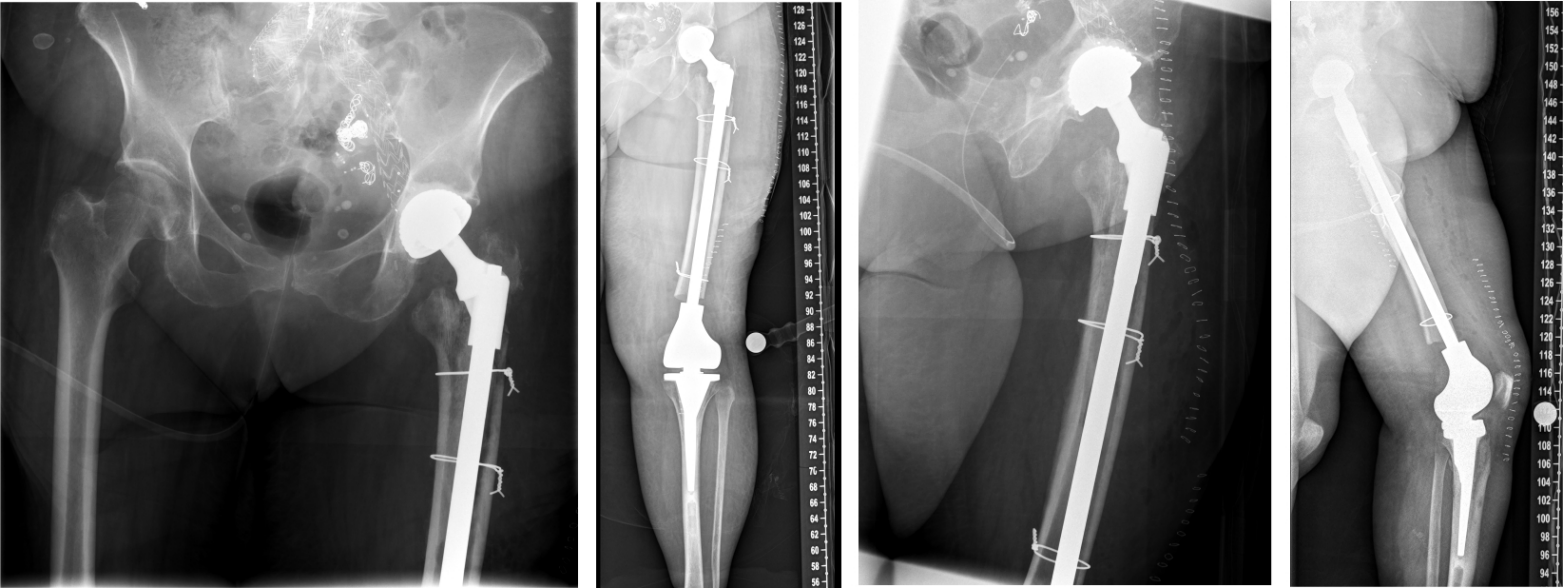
The postoperative radiographs showed correct implant position and a satisfactory surgical result.
